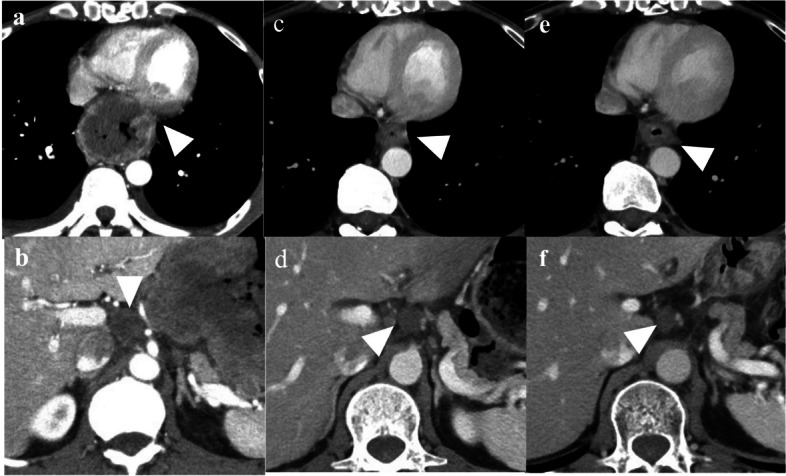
Enhanced CT findings. a CT images showing a large mass at the esophageal junction. Tumor invasion into the left atrium was suspected (white arrow head). b Intra-abdominal lymph node metastases were identified (white arrow head). c Enhanced CT images after two courses of chemotherapy showing that the primary tumor has decreased in size (white arrow head). d The lymph node metastases remain shrunk (white arrow head). e Enhanced CT images after four courses of chemotherapy showing that the primary tumor has enlarged slightly (white arrow head). f The lymph node metastases have also decreased in size (white arrow head)
